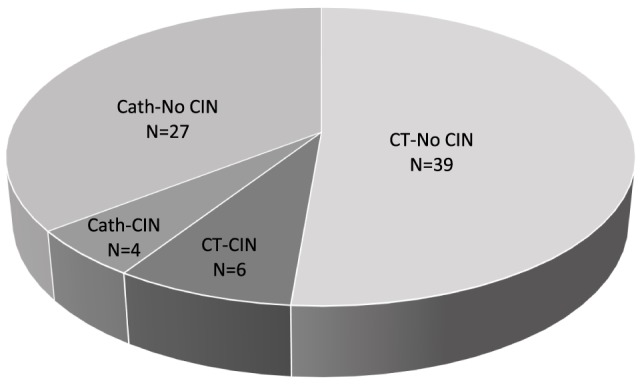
Contrast-induced nephropathy (CIN) frequency. Total number (*N*) of contrast media (CM) exposures = 76, *N* of CM exposures *via* computed tomography (CT) = 45, *N* of CM exposures *via* coronary angiogram (Cath) = 31.
